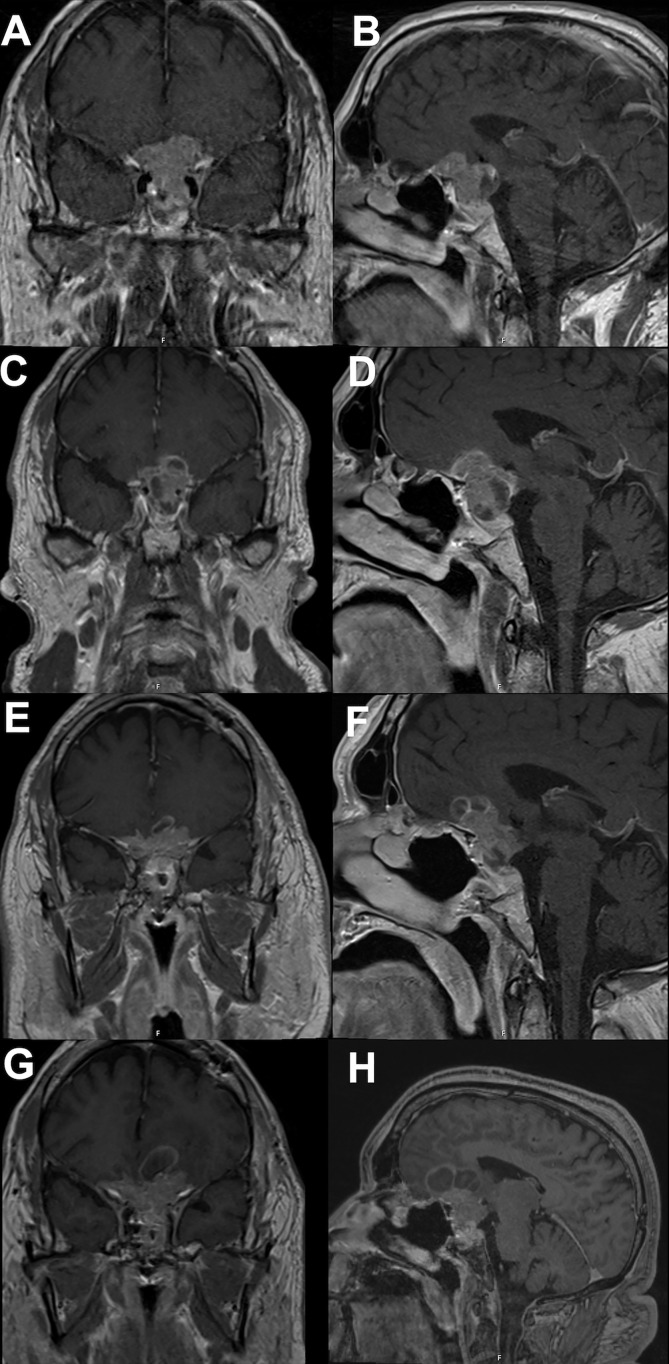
Brain MRI showing tumor progression. (A coronal; B sagittal) showing continued tumor progression after temozolomide therapy and prior to second operation. (C coronal; D sagittal) showing tumor prior to pazopanib therapy. (E coronal; F sagittal) showing tumor response to pazopanib therapy, tumor size slightly decreased and stabilized for 6 months. (G coronal; H sagittal) showing continued tumor progression despite ongoing therapy.
